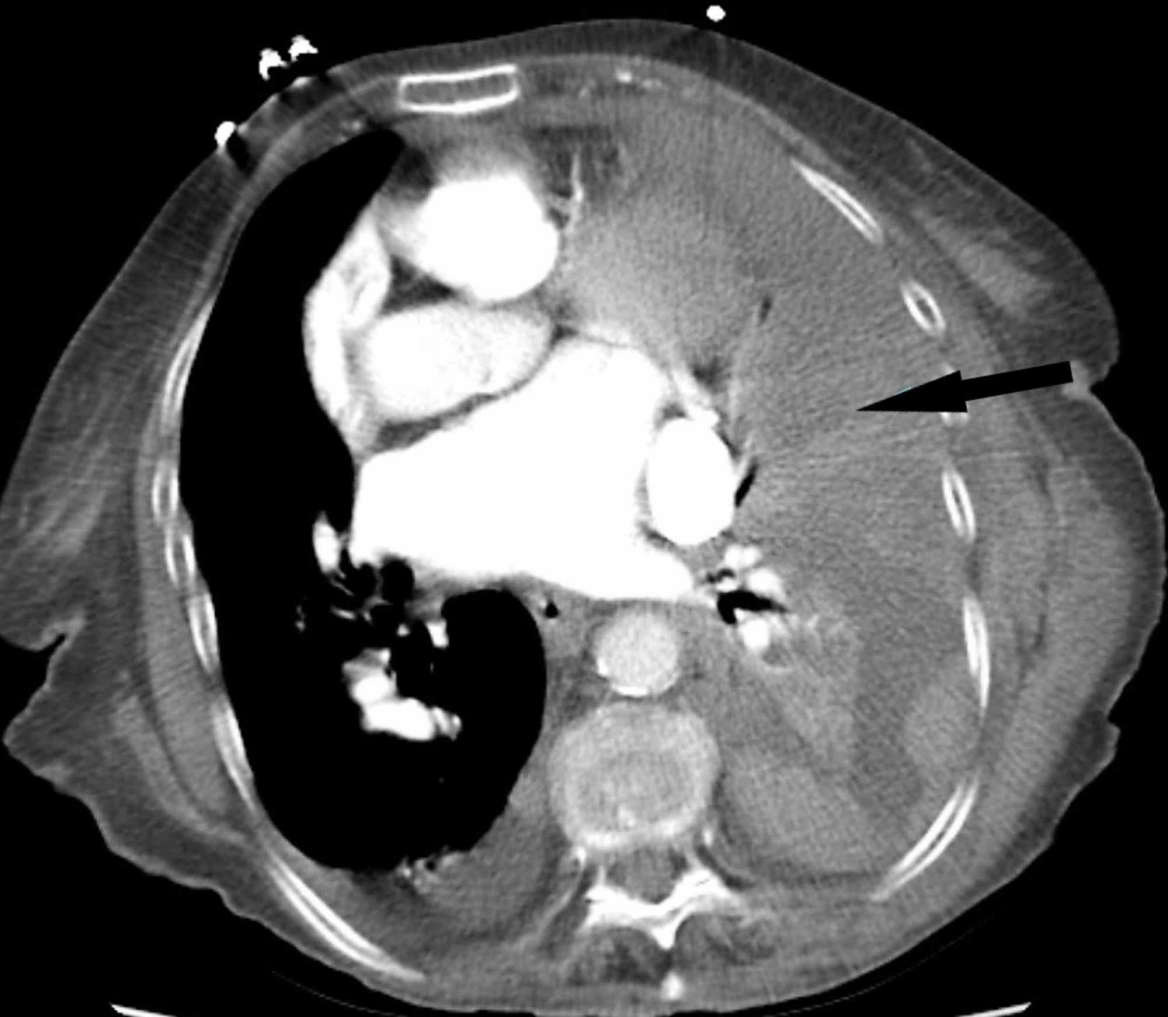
CT axial plane images section from prior to the placement of chest tube
